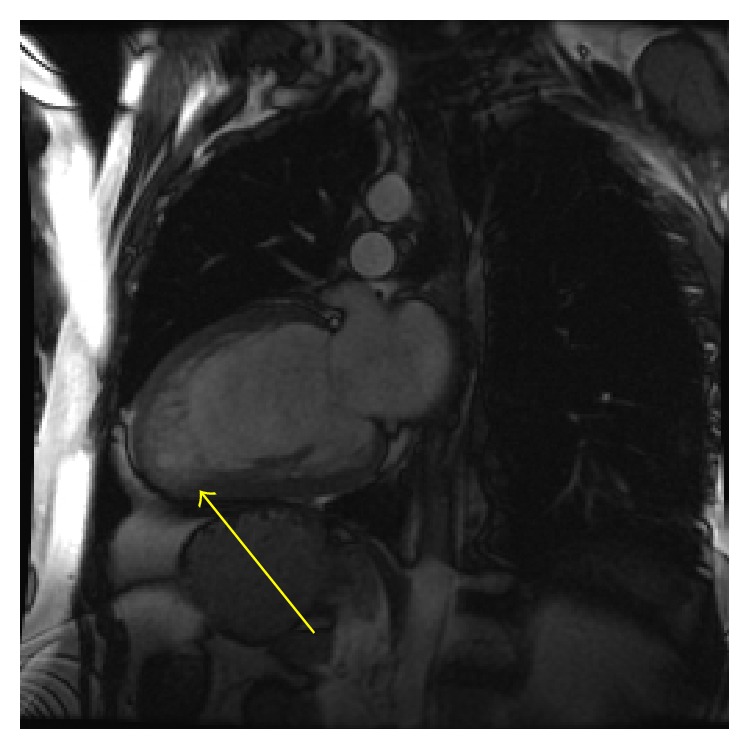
Cardiac magnetic resonance imaging (CMR) of a 38-year-old male that presented with a new cardiomyopathy and congestive heart failure. Note the prominent apical trabeculations compared to the compacted myocardium. Cardiac catheterization demonstrated no angiographic coronary disease and LVNC was the most likely diagnosis.
